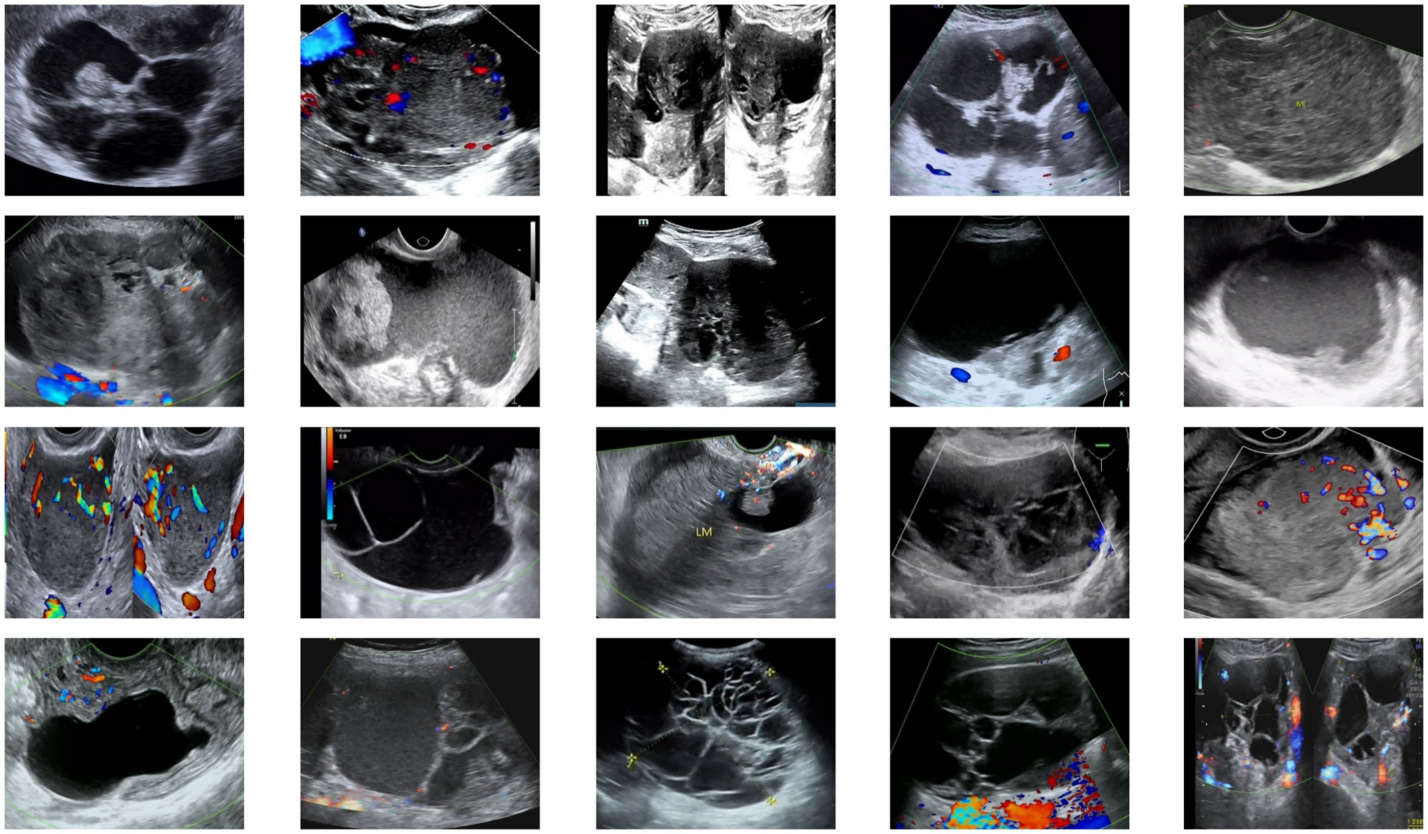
Examples from the ovarian tumor ultrasound dataset.
